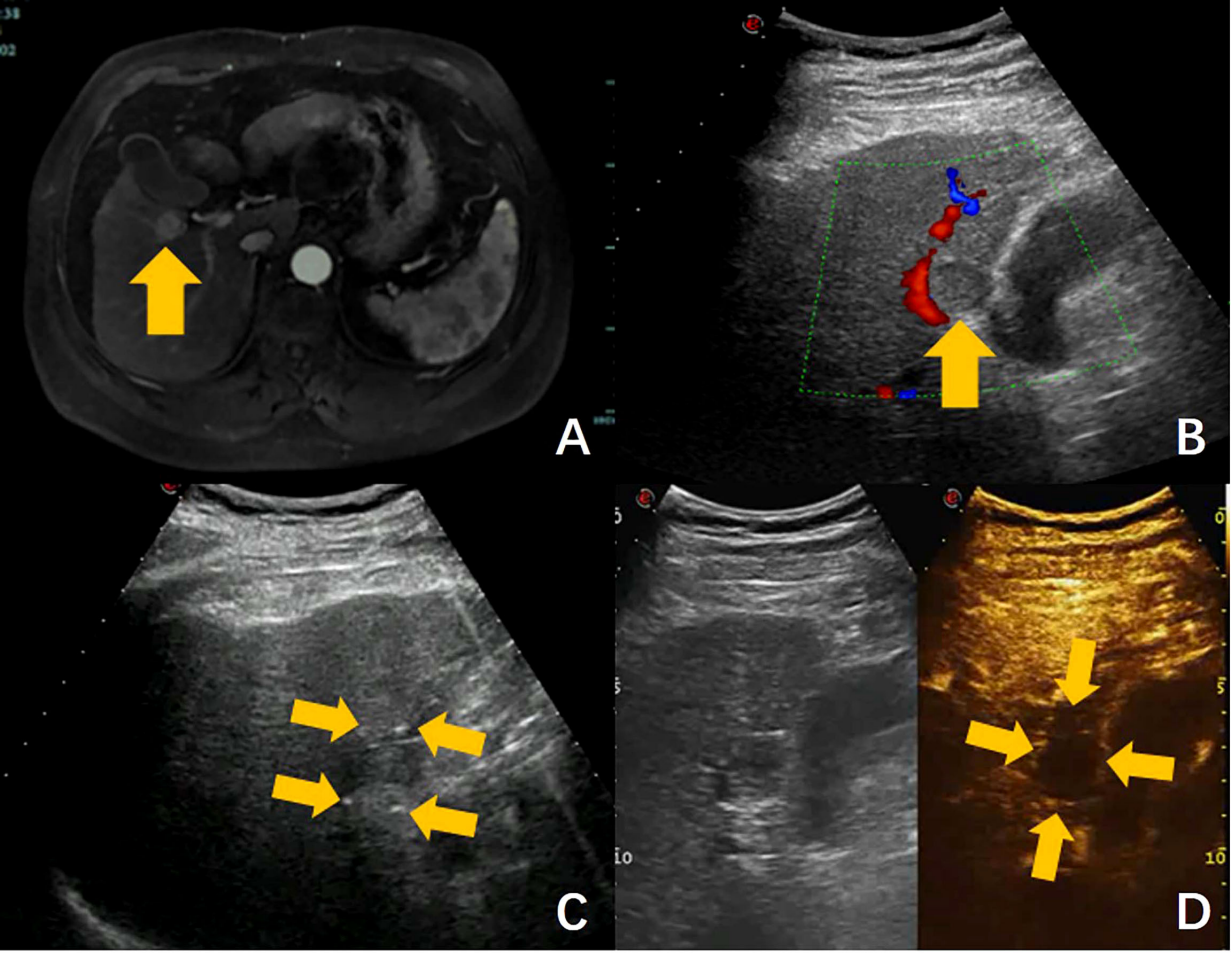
Ultrasound-guided nsPEF procedure for HCC adjacent to gallbladder. (A) Pre-ablation contrast-enhanced MRI revealed a hepatocellular carcinoma adjacent to gallbladder. (B) Ultrasound showed a hypoecho tumor located in close contact with gallbladder and right portal branch (arrow). (C) Ultrasound-guided nsPEF with a two-electrode configuration, and the head and tail end of the active tip of the electrodes can have punctuated enhancements (arrow). (D) The contrast-enhanced ultrasound image revealed no enhancement after ablation. (arrow).
